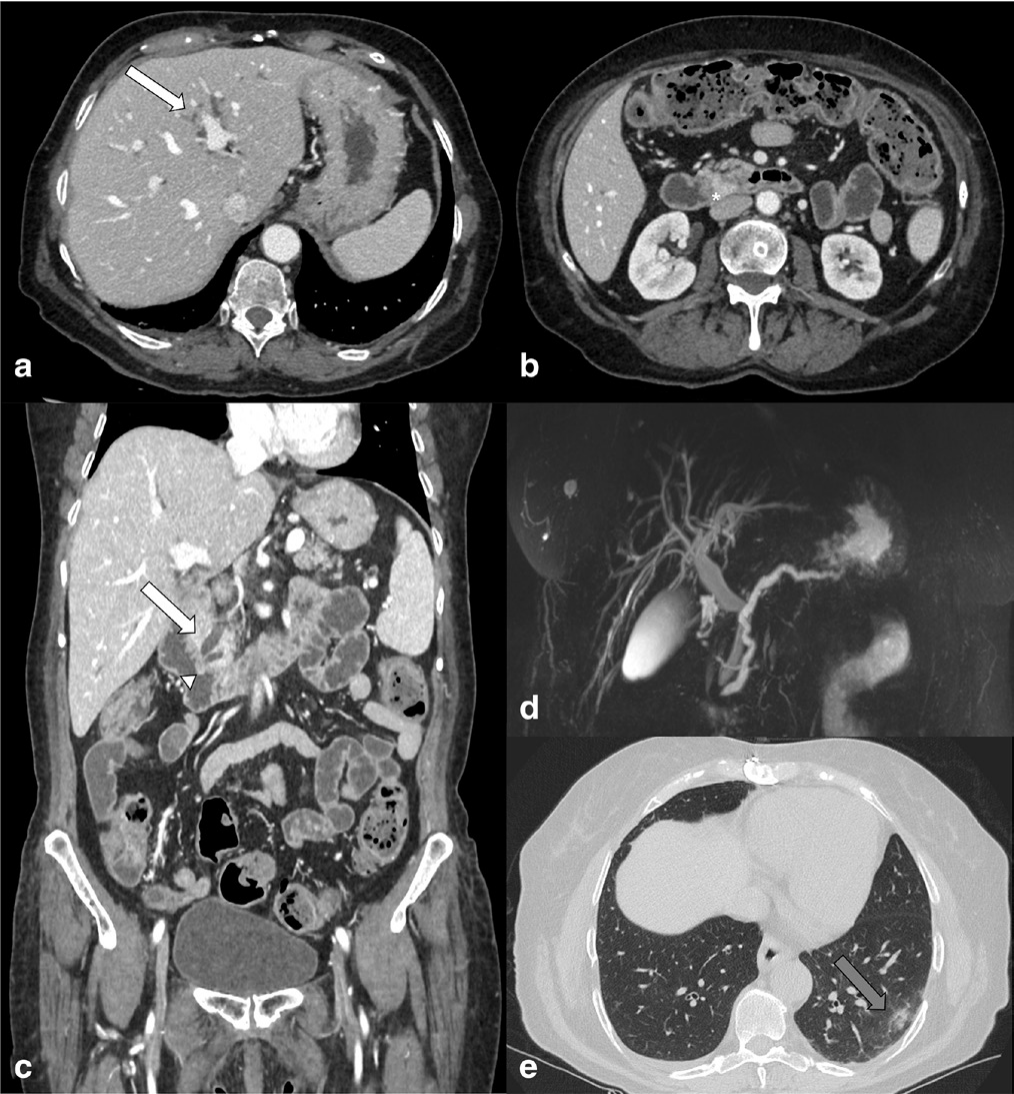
Enhanced CT scan (portal phase) of the abdomen of a 67-yo female admitted to
